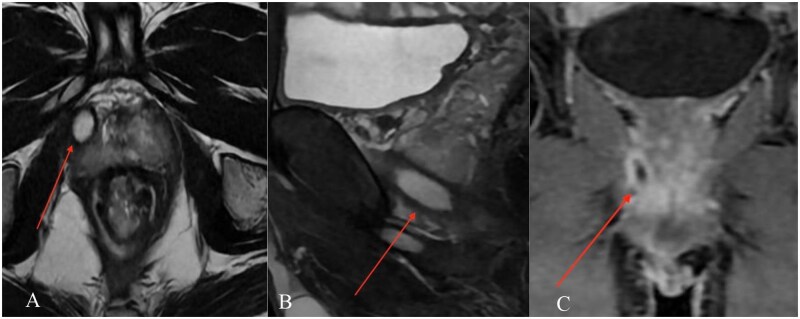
Additional right-sided extension of the fistulous disease. (A) Axial T2-weighted image shows a rounded hyperintense right perianal/perirectal collection or tract component (arrow). (B) Sagittal T2-weighted image demonstrates cranial extension of the abnormal tract/collection along the right pelvic sidewall toward the inferior periprostatic region (arrow). (C) Coronal post-contrast fat-suppressed T1-weighted image shows enhancement around the right-sided tract/collection, supporting active inflammatory change (arrow).
